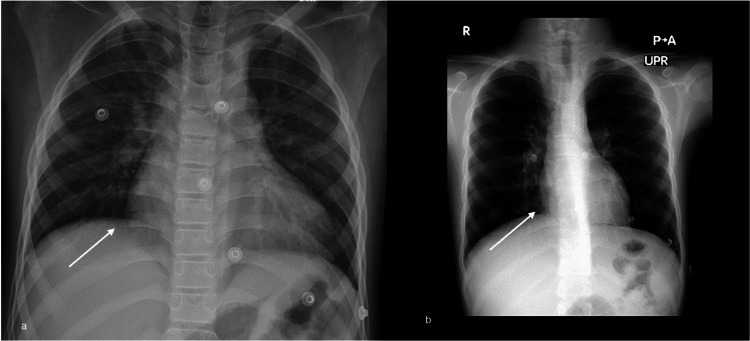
Chest X-ray (a) Anteroposterior chest radiograph shows cardiomegaly (b) After coarctation stenting
